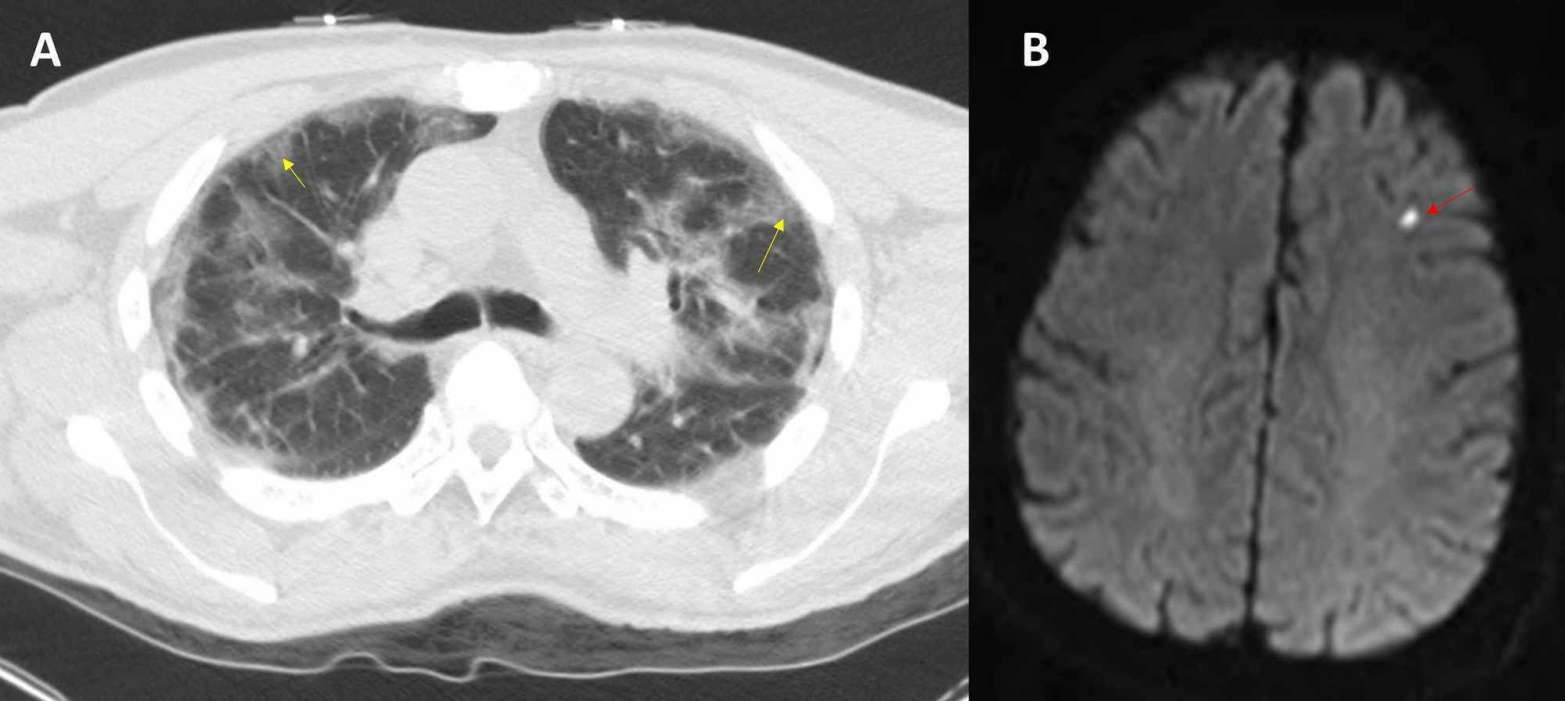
CT of the chest and MRI of the brain. A: CT of the chest showing bilateral peripheral ground glass opacities (yellow arrow). B: MRI of the brain showing an acute infarct in the left frontal lobe (red arrow).
